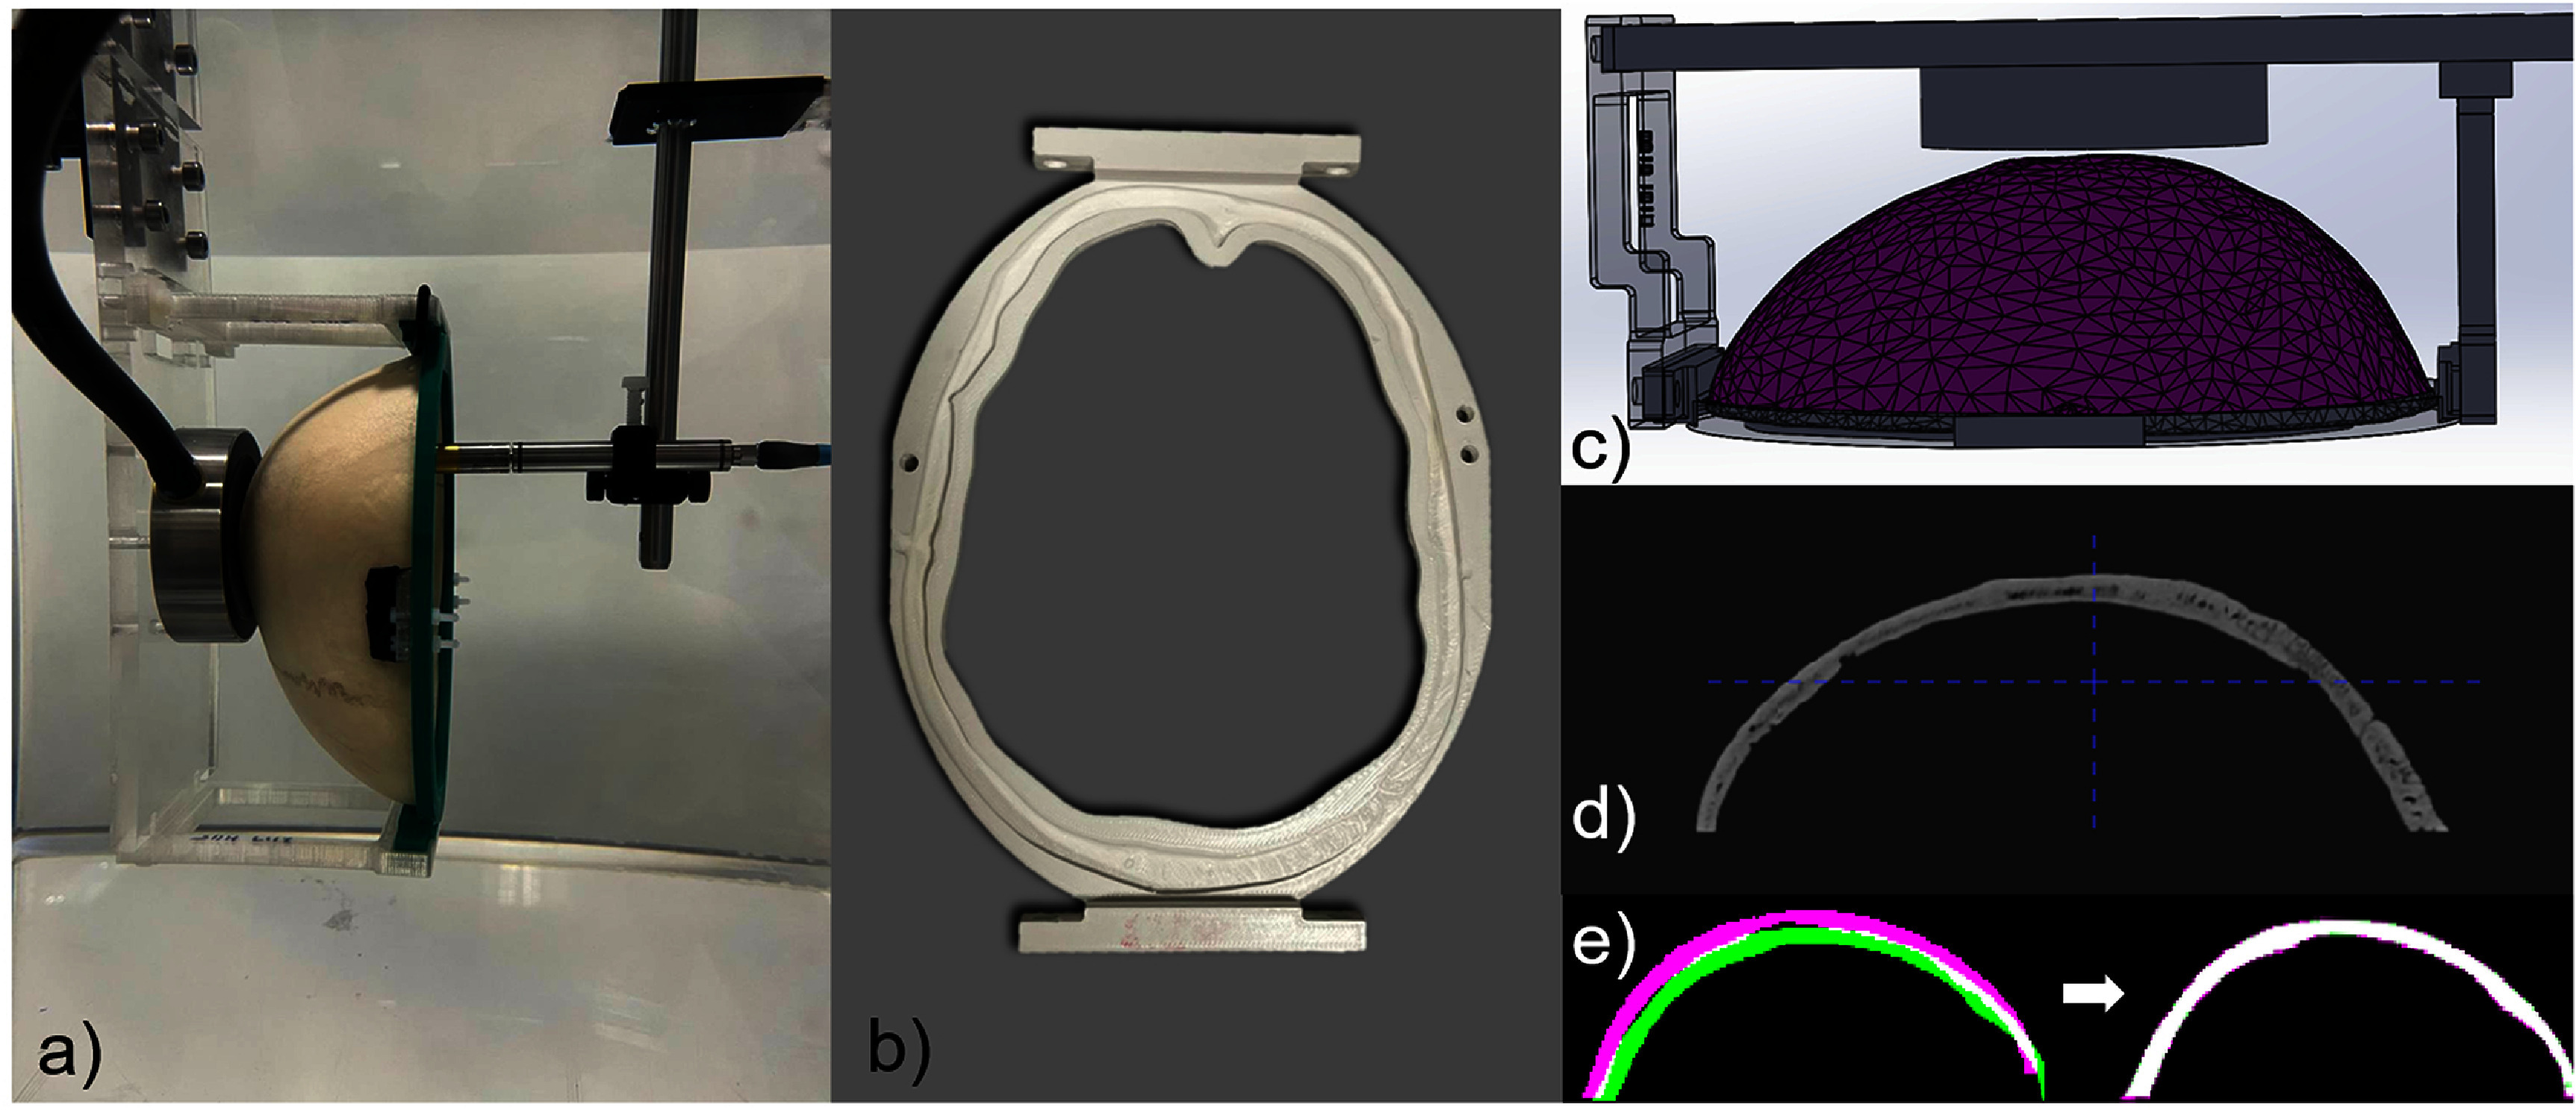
(a) Experimental setup showing hydrophone scanning within the cavity of an *ex-vivo* skull calvarium which is held in position by a (b) 3D-printed plastic skull holder and mounts attached to the transducer mounting plate; (c) 3D CAD model of the experimental setup; (d) CT image of a skull; (e) CT image mask and skull mesh registration.
